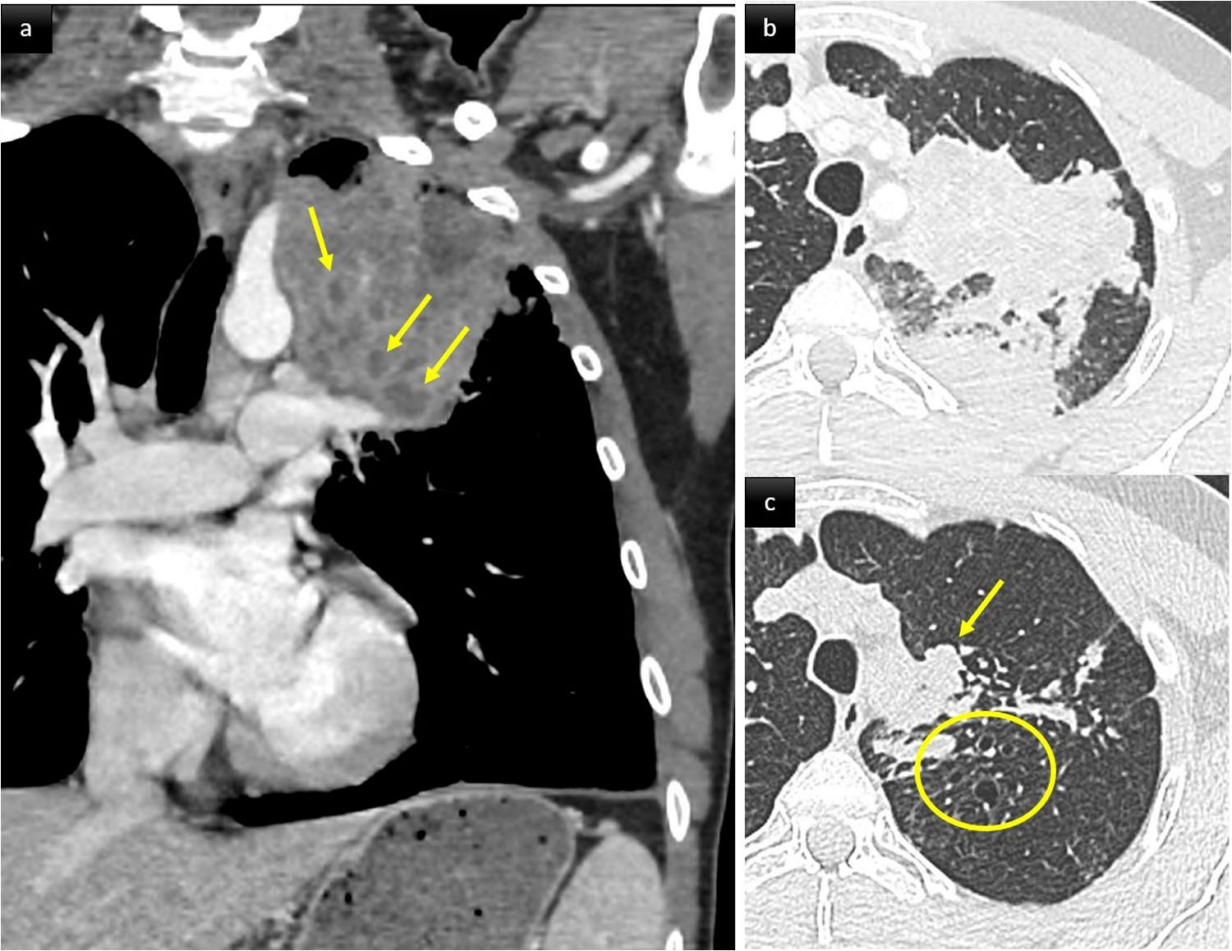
Chronic granulomatous disease (CGD) revealed by a mixed pattern of aspergillosis in a 41 yo man. Coronal post contrast (a) and axial parenchymal (b, c) CT images. Large partially necrotic consolidation in the left upper lobe. Tubular opacities less dense than airspace consolidation are consistent with plugged dilated airways (arrows). Note the presence of underlying varicose bronchiectasis in the same lobe (circle)
